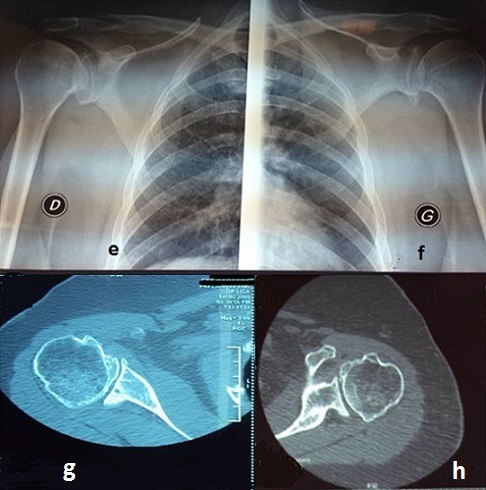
(e,f) la radiographie standard a objectivé une omarthrose avancée de l'articulation glèno-humérale classé stade V; (g,h) un complément scannographique de l’épaule bidimensionnel (2D) et tridimensionnel (3D)
